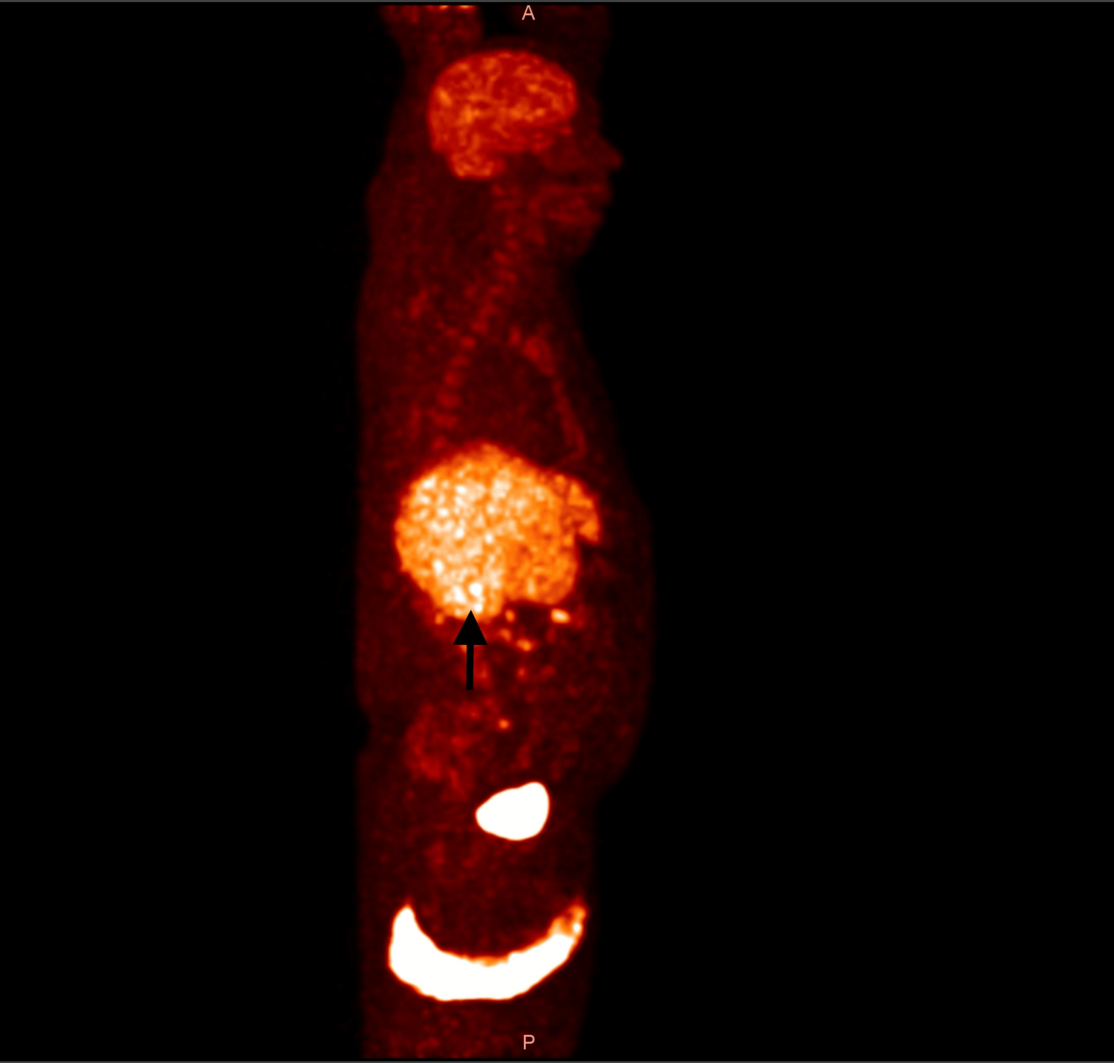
Whole-body PET-CT (sagittal view) Sagittal PET-CT image showing diffuse hepatic and splenic FDG uptake, consistent with inflammatory changes and absence of discrete masses. FDG, fluorodeoxyglucose
